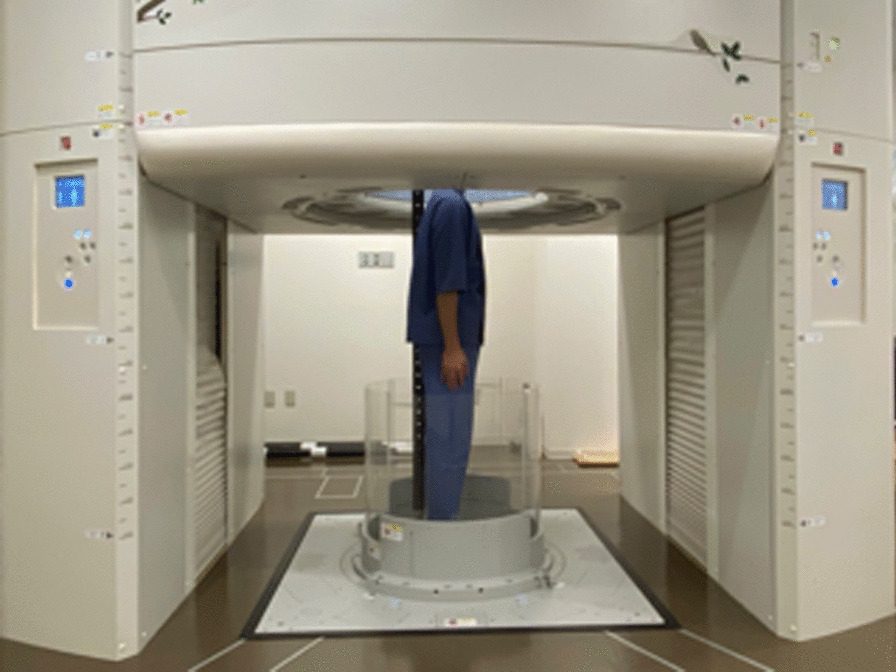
Computed tomography (CT) images of the bilateral upper extremities were obtained in the standing neutral position using an upright CT scanner
